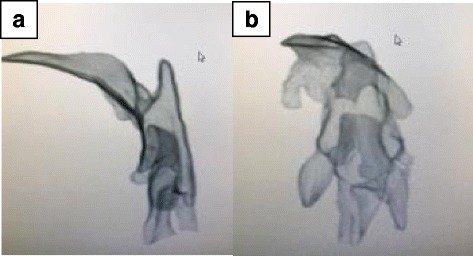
Postoperative 3D CT image of the upper airway. a Lateral view of the upper airway on 3D CT. b On a dorsal view, circumflex of the upper airway was noted
